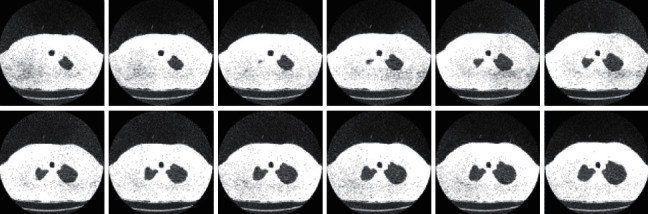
Sample of dataset CT-scan images.
